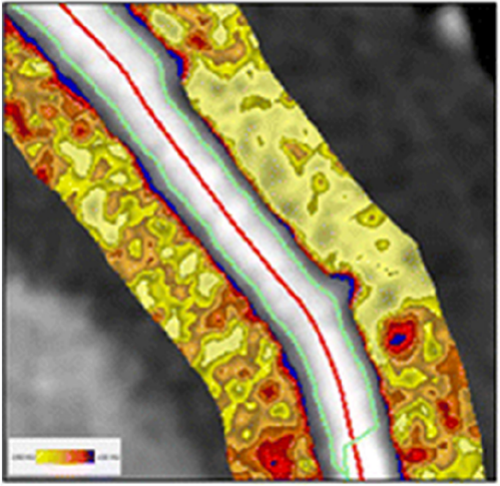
## Optimal trial design: learnings from recent serial coronary CT angiography studies
